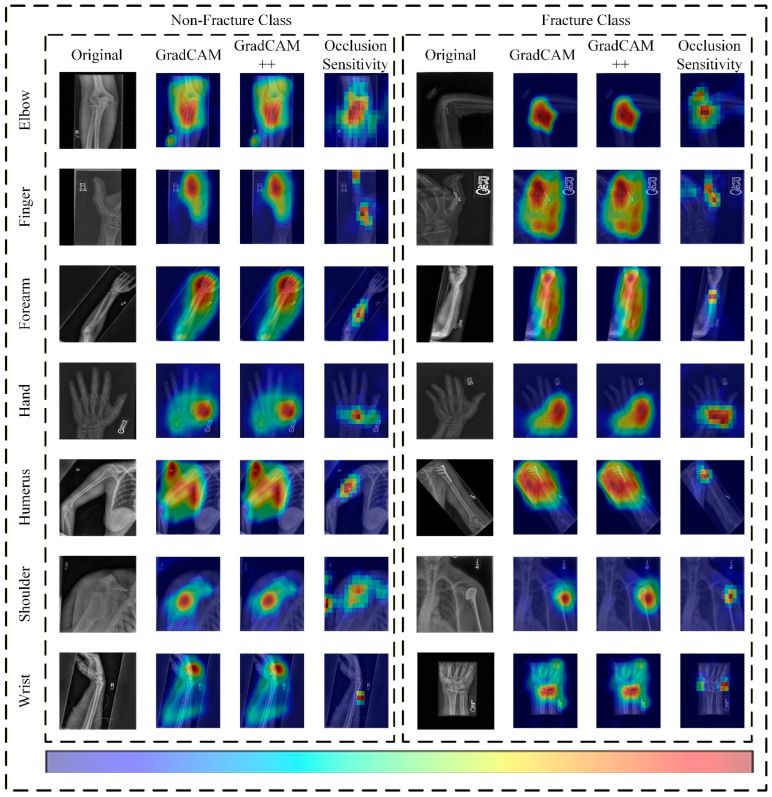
Representative explainability results for the proposed Xception-Swin model across non-fracture and fracture classes for elbow, finger, forearm, hand, humerus, shoulder, and wrist, showing the original radiographs together with Grad-CAM, Grad-CAM++, and occlusion sensitivity maps that highlight the anatomically relevant regions contributing to the model’s predictions. Warmer colors, including yellow, orange, and red, indicate regions with higher model attention or stronger contribution to the prediction, whereas cooler colors, including blue and green, indicate regions with lower model attention or weaker contribution.
